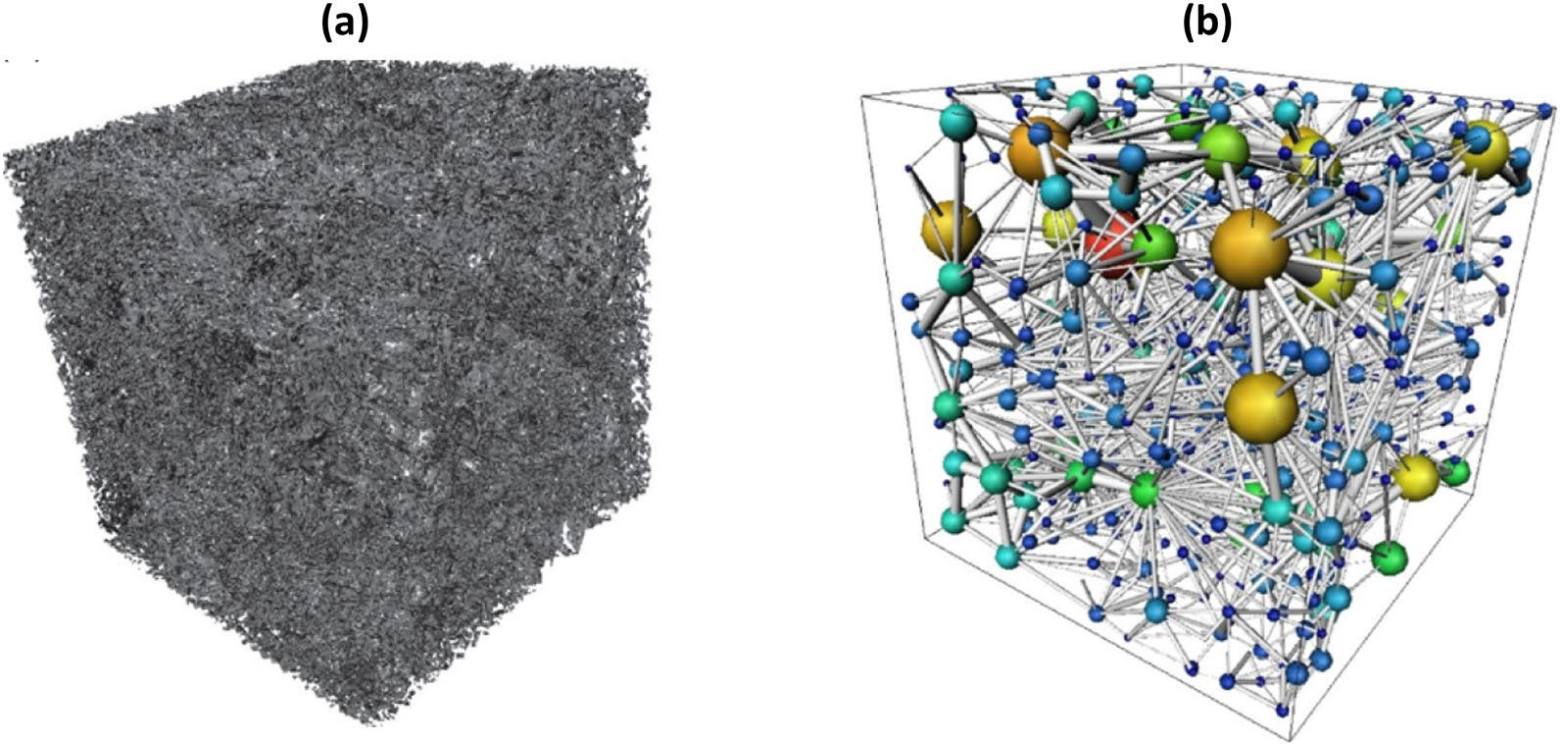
a Pore-space image of Mount Gambier; b its pore-network model extracted/computed from CT images [60]
